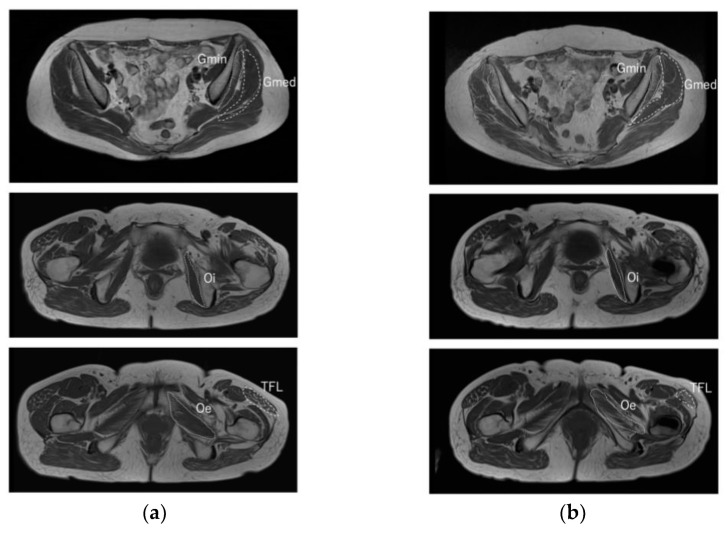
Pre-operative and 12-month post-operative T_1_-weighted magnetic resonance imaging axial scans of the bilateral hip joint. (a,b) show pre-operative and 12-month post-operative images, respectively. The left side is the affected side. Muscles surrounding the left hip joint analyzed were the Gmin (solid line), the Gmed (dashed line), the Oi (solid line), the Oe (dotted line), and the tensor fascia latae (TFL) (dashed line). Post-operative TFL shows decreased muscle cross-sectional area and worsened fatty atrophy.
